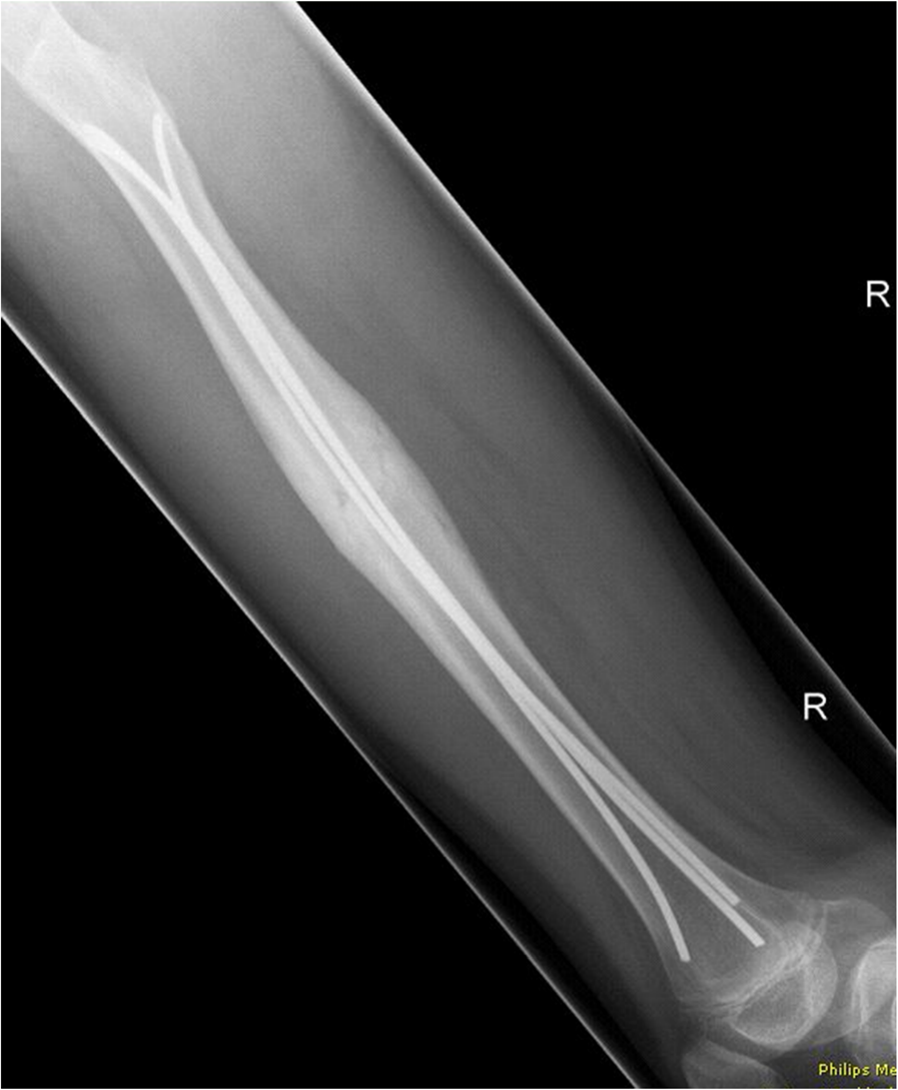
Radiograph (lateral view) of a 3CM configuration, 4 months postoperativly, diameter of the nails: 3 × 3.5 mm.
